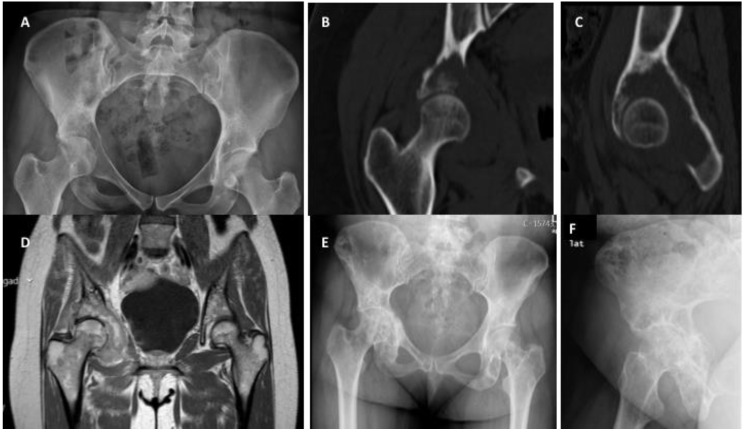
Right acetabular impending fracture in a female patient with breast cancer and multimetastatic bone disease: (A) anteroposterior pelvic radiograph showing a lytic lesion located in the right acetabulum, (B) pelvic coronal computed tomography showing in more detail the lytic bone metastasis on the right acetabulum, visible in plain radiograph, (C) sagittal computed tomography showing a detailed characterization of the lytic bone metastasis on the right acetabulum, (D) magnetic resonance coronal image showing the extension of breast cancer metastasis, occupying the right acetabulum, (E) pelvic anteroposterior radiograph showing an exceptional result three months after radiation and denosumab treatment of the impending fracture in (A), (F) alar view of the right acetabulum after radiation and denosumab treatment.
